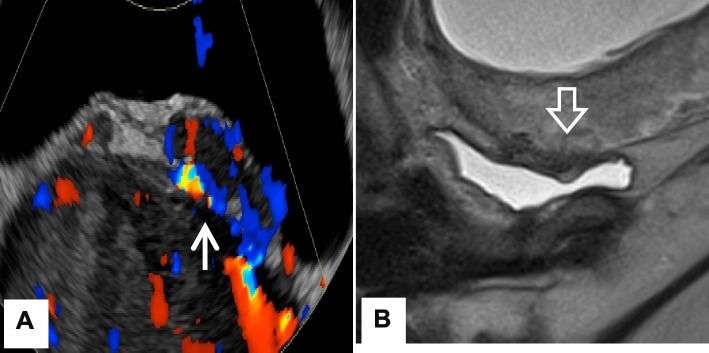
Antenatally suspected placenta percreta with A transvaginal ultrasound demonstrating myometrial thinning with several tortuous vessels (←) between the uterus and bladder, and B T2-weighted coronal MRI demonstrating thinning of myometrium and superior bladder wall irregularity(⇦) without definite bladder wall invasion
